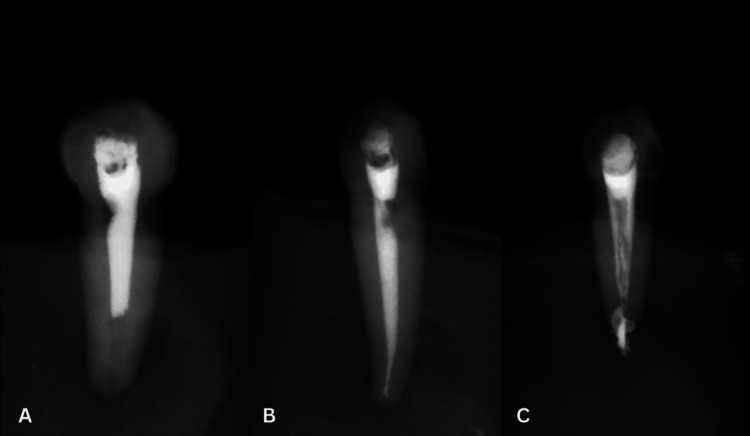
Radiographs showing the obturation's length A. Underfilling. B. Optimal filling. C. Overfilling
